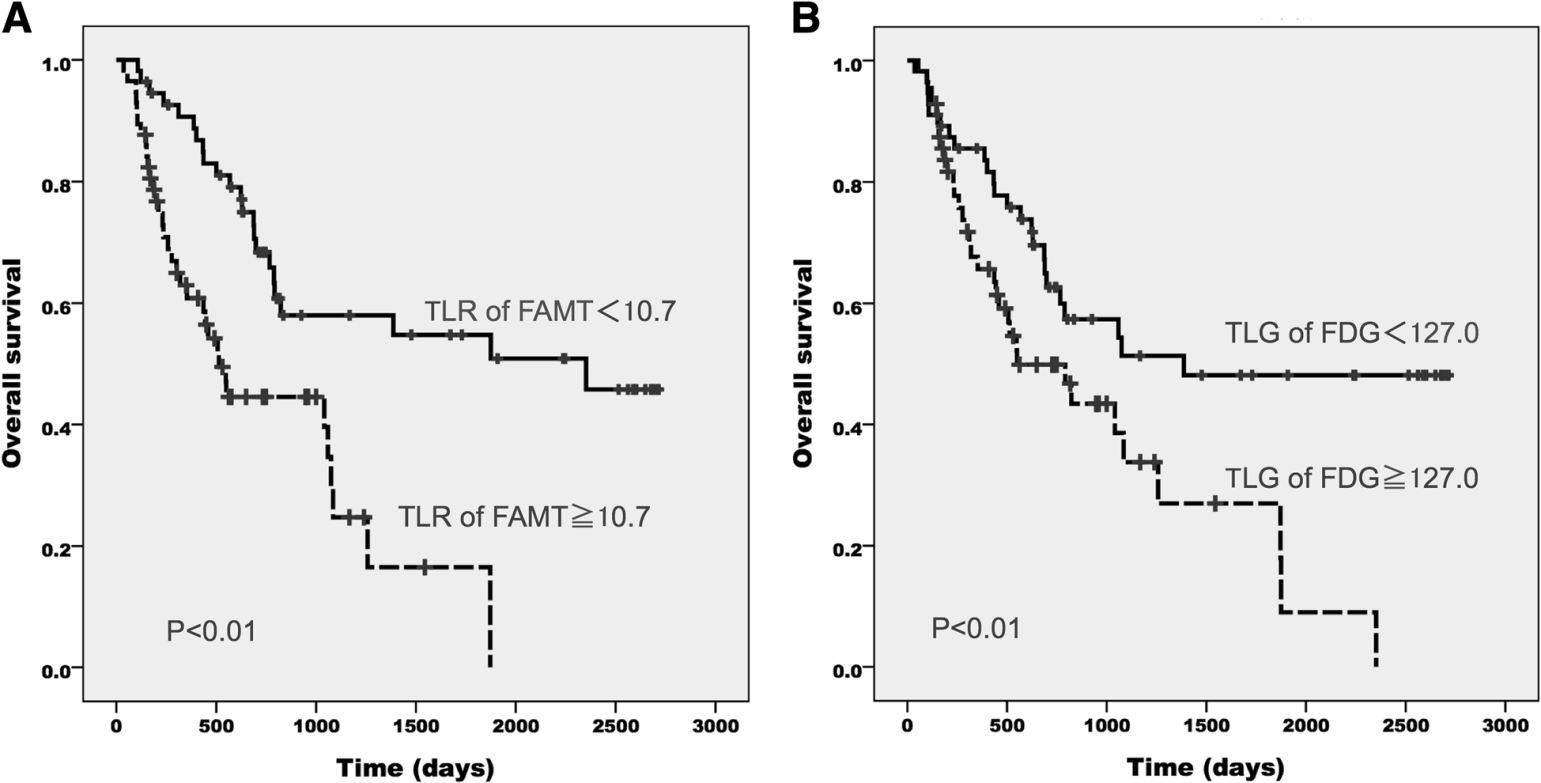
Overall survival of NSCLC patients according to the TLR of ^18^F-FAMT (a) and TLG of ^18^F-FDG (b)
